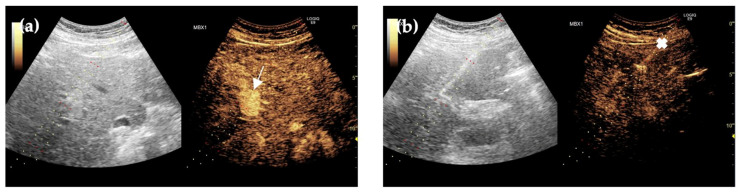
Forty-six months after first imaging, CEUS demonstrated contrast accumulation in the porto-venous phase (arrow) (a) and a biopsy needle (cross) positioned in the desired localization (b). On B-mode ultrasound, the lesion appears predominantly hypoechoic with possible internal thin septations.
